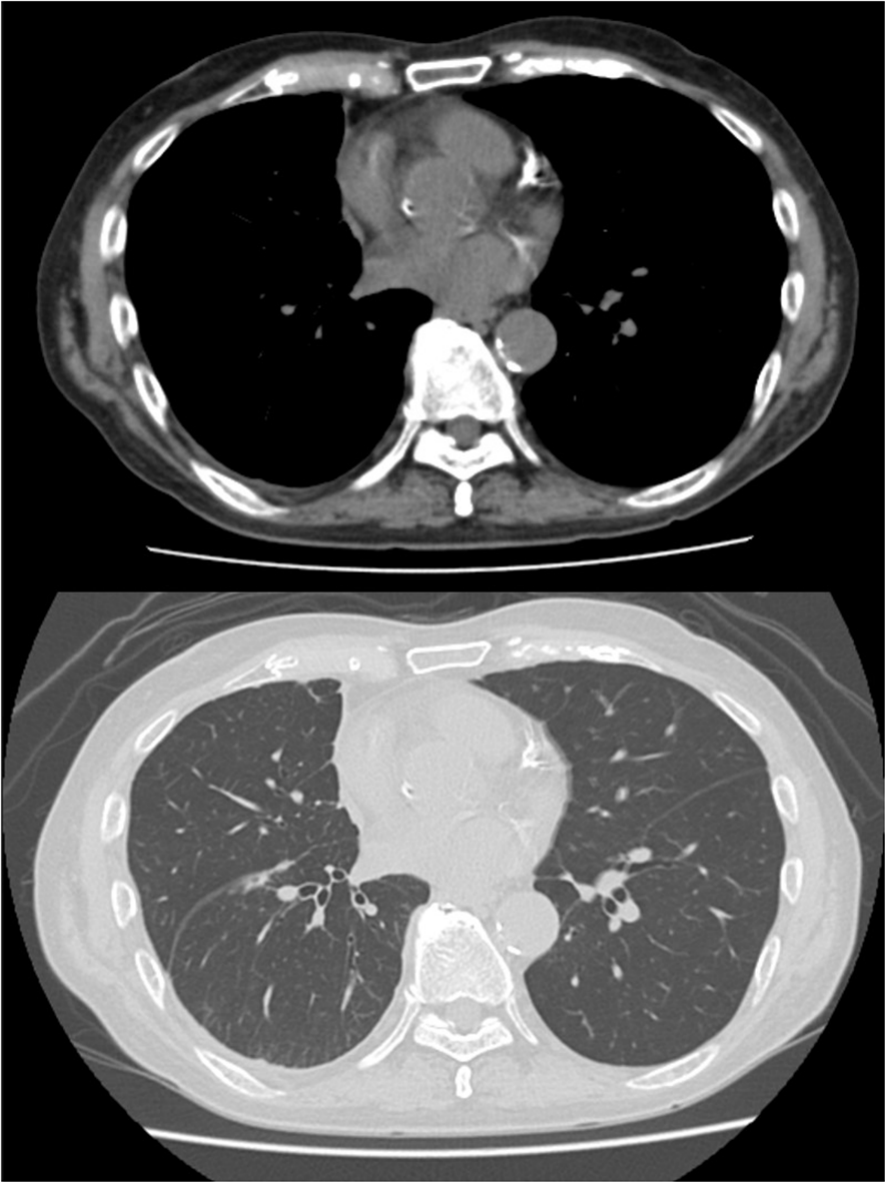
Radiographic response to a single cycle of immunotherapy. CT scans after one cycle of nivolumab and ipilimumab, demonstrating a significant reduction in the solid tumor lesions (white arrows) and complete resolution of the pleural effusion. CRP, C-reactive protein; CRS, cytokine release syndrome; ICI, immune checkpoint inhibitor; IL-6, interleukin-6; irAE, immune-related adverse event; mPSL, methylprednisolone; PSL, prednisolone.
